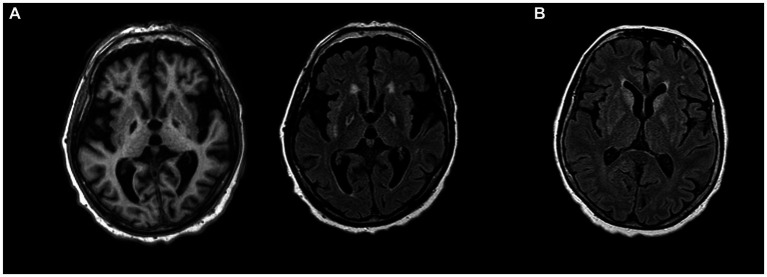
(A) T1-weighted hypointensities (left) and T2-weighted hyperintensities (right) bilaterally in globus pallidus in a patient with parkinsonism and cognitive decline after carbon monoxide posioning. (B) FLAIR hyperintensities in bilateral basal ganglia in anti-Ma2 encephalitis presenting with parkinsonism.
